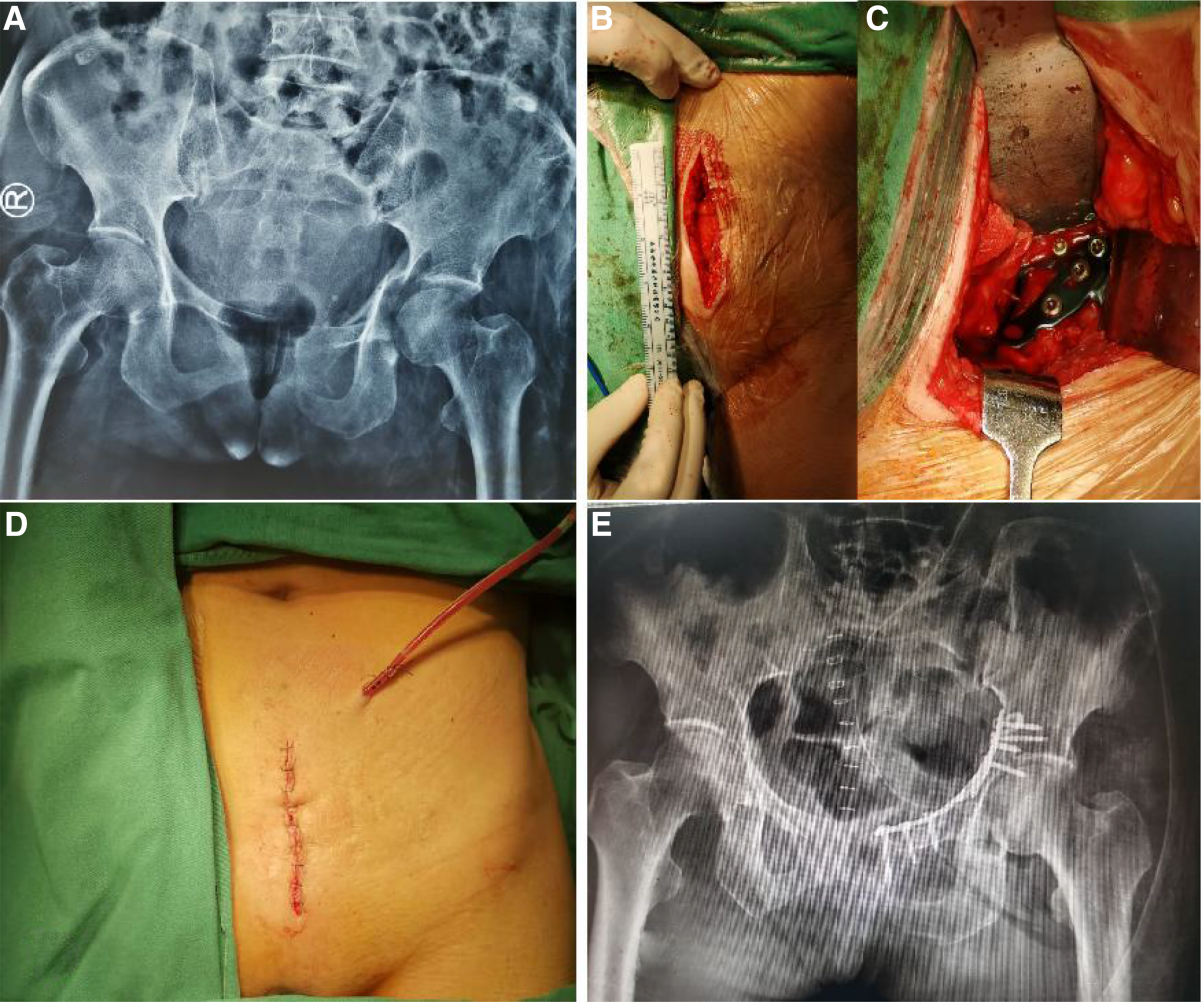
A female patient, 57 years old, with a T-type acetabular fracture who underwent surgical treatment *via* a modified stoppa approach. (A) preoperative anteroposterior pelvic radiograph; (B), picture of the incision of the modified Stoppa approach; (C), internal fixation was placed after fracture reduction; (D), a CSD was used after surgery; (E), postoperative anteroposterior pelvic radiograph.
